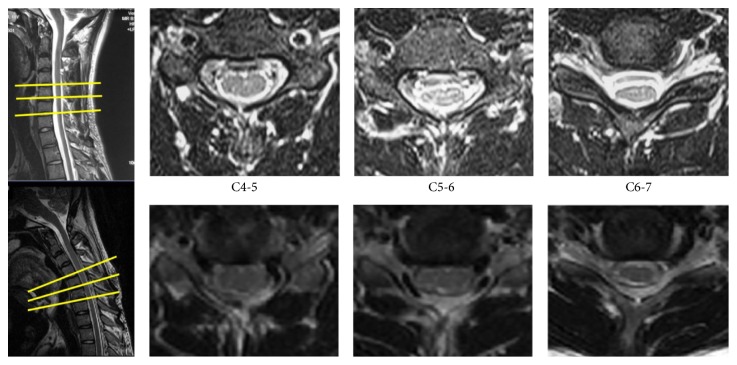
A case of 18-year-old man with Hirayama disease: T2-weighted sagittal and axial images showing a significant forward displacement of the spinal cord after cervical flexion movement and an increase in abnormal signal posterior to the dural sac.
